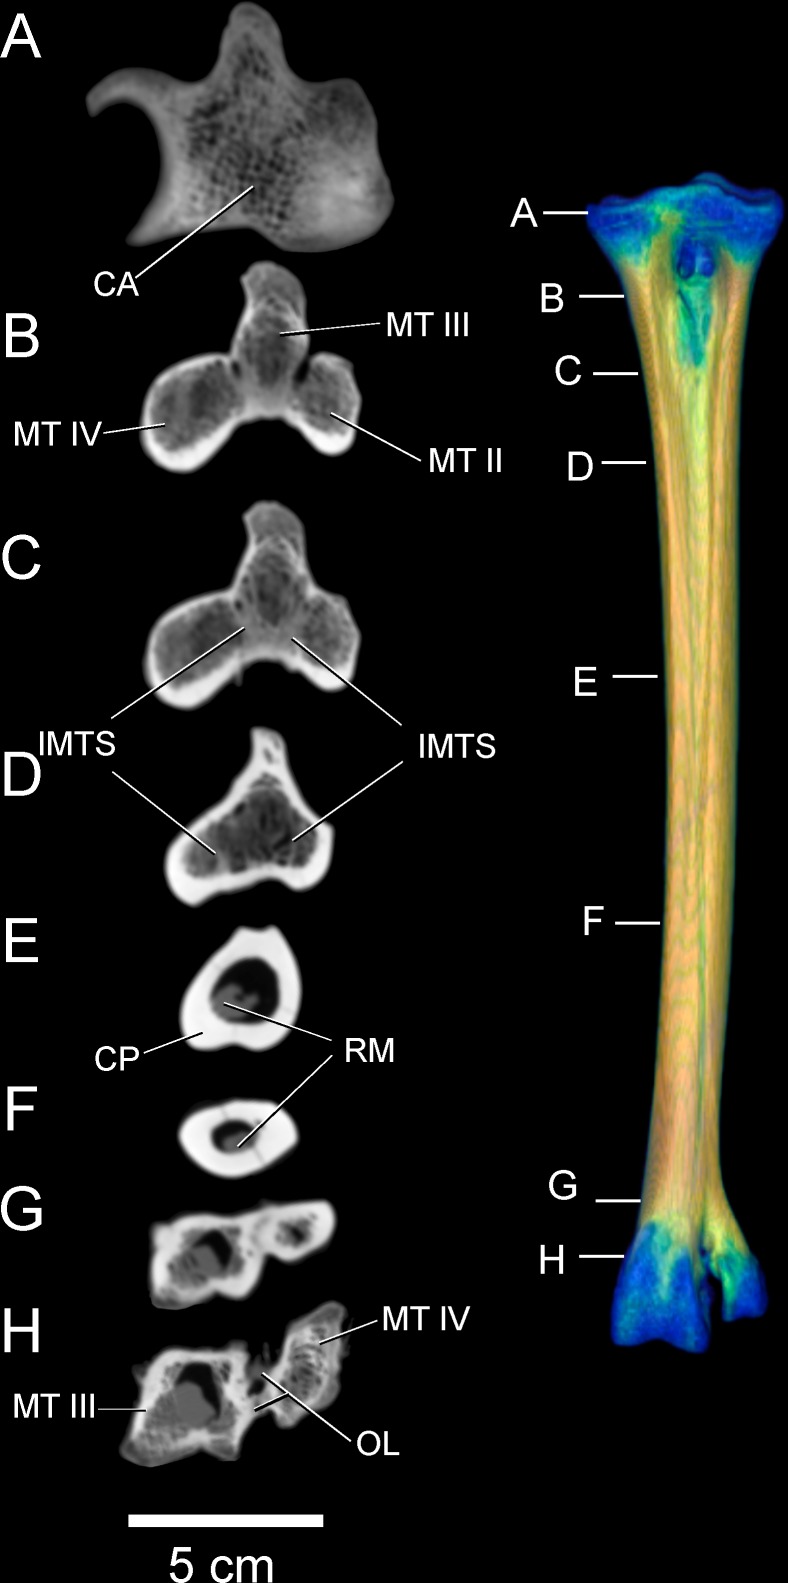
CT cross sections of left adult tarsometatarsus, from proximal (A) to distal (H). Despite ankylosis, distinct metatarsal bones separated by intermetatarsal septa are present in the proximal and distal sections of the bone. The individual metatarsals become fully fused through the center of the shaft, creating a circular, hollow tube composed of compact bone. Abbreviations: CA = cancellous bone, CP = compact bone, IMTS = intermetatarsal septum, MT II = metatarsal II, MT III = metatarsal III, MT IV = metatarsal IV, OL = ossified ligament, RM = residual marrow.
